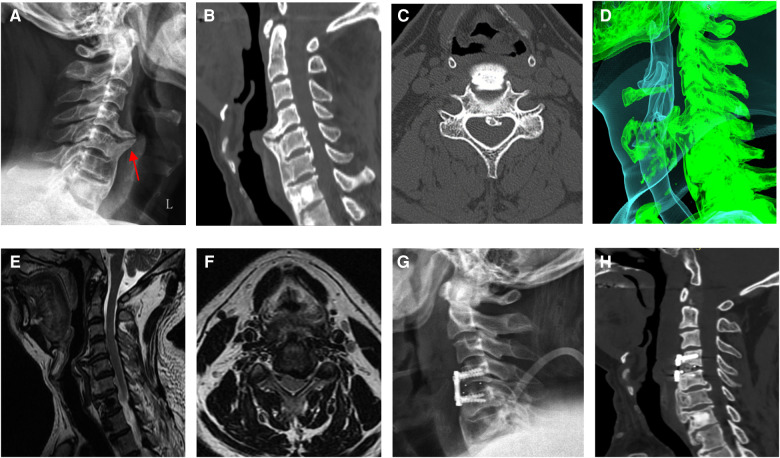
(A) osteophyte formation can be seen in front of the C4–C7 vertebral body on cervical radiographs before operation, and a giant coracoid osteophyte can be seen at the anterior edge of C4–C5. (B,C) The C4–C5 segment showed the formation of a huge osteophyte in the anterior margin and ossification of the posterior longitudinal ligament in the spinal canal. (D) Preoperative three-dimensional computed tomography (CT) reconstruction showing that hyperplastic osteophytes compressed the trachea and esophagus. (E,F) Preoperative magnetic resonance imaging showing that the spinal canal space became narrower at the C4–C5 level. (G,H) Radiographic and CT examination after operation showed that the large osteophyte in front of the C4-C5 vertebral body had been removed.
